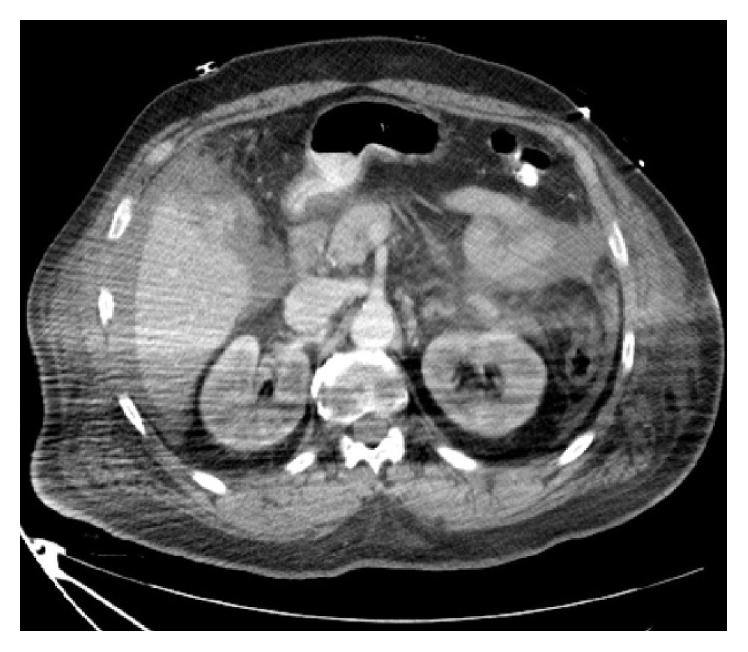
CT scan of abdomen and pelvic showing hemoperitoneum and mass-like density abutting gallbladder.
